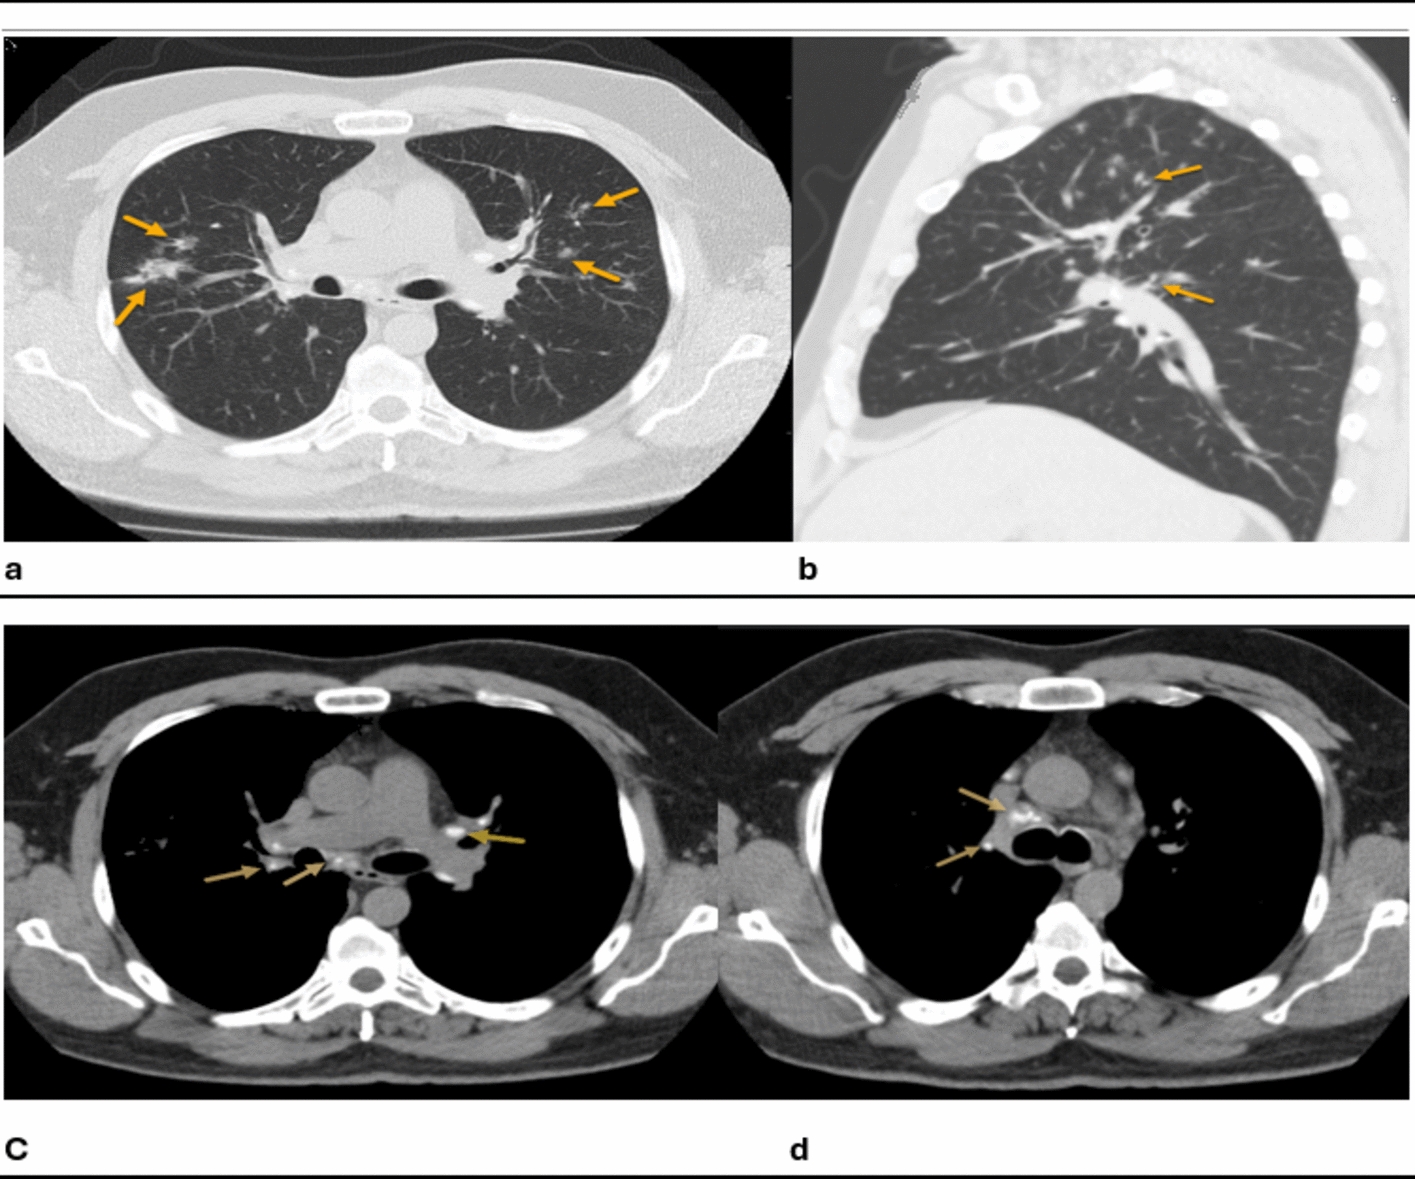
Computed tomography scan of the chest demonstrates radiological features consistent with pulmonary sarcoidosis. Axial (a) and sagittal (b) chest computed tomography scans with lung window demonstrate diffuse patchy perilymphatic nodules and opacities bilaterally, most notable in the upper lung lobes (the four yellow arrows in image a and the two yellow arrows in image b). The axial chest computed tomography with mediastinal window (c, d) shows partially calcified mediastinal and hilar lymph nodes (the three yellow arrows in image c and the two yellow arrows in image d)
